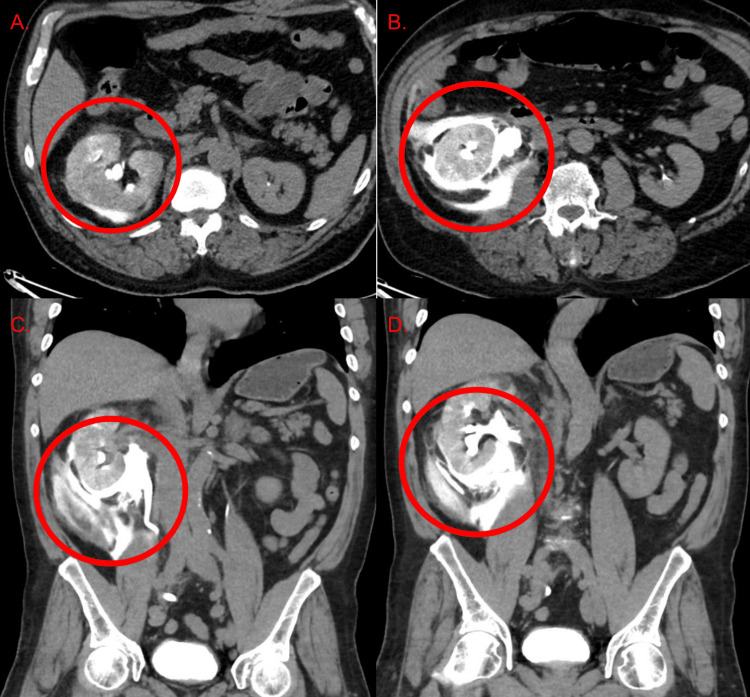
Panels A-D demonstrate the extravasation of contrast media on the right-sided renal collecting system on a delayed, non-contrast computed tomography scan (red circles). This figure demonstrates the importance of delayed imaging in the setting of forniceal rupture because it allows the contrast media sufficient time to be filtered by the kidney and subsequently travel into the collecting system to depict the location and extent of the urinary extravasation.
